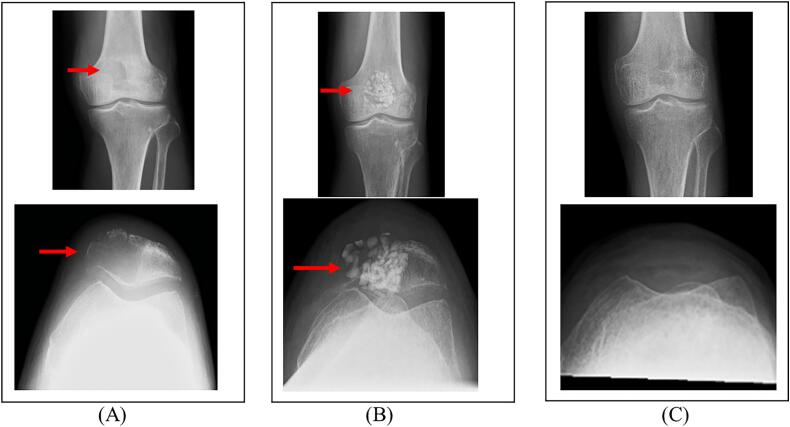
Preoperative and postoperative radiographs of the left knee of a 65-year-old man. (A) Preoperative frontal and lateral views of the patella showing osteolysis (arrowhead). (B) Frontal and lateral views after curettage of the tumor and grafting of the artificial and autologous bones (arrowhead). (C) Frontal and lateral views after patellectomy.
